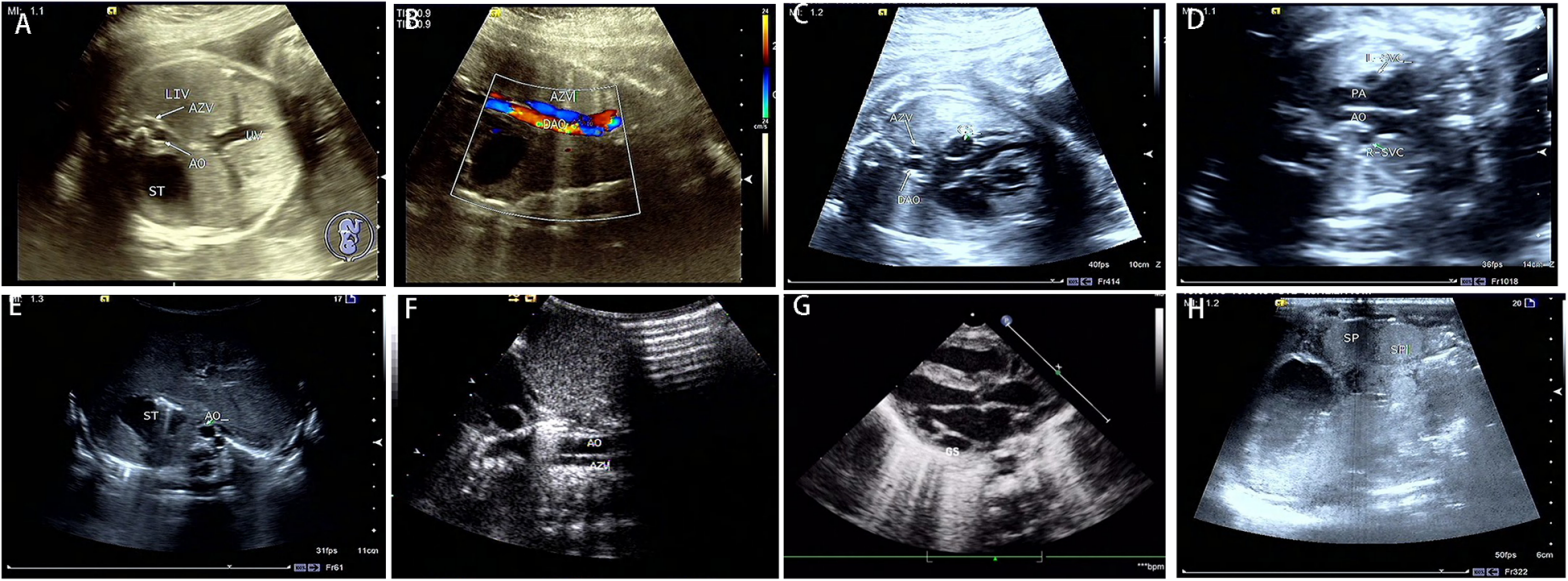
Prenatal and postnatal ultrasonography of LI fetuses. Prenatal sonography in the transverse section of the abdomen (A–D). (A) absence of a hepatic segment of IVC with dilated azygos; ST gastric vesicle, AO, abdominal aorta, AV azygos vein, and UV umbilical vein. (B) the parallel arrangement of the azygos vein and descending aorta. DAO, descending aorta. (C) the dilatation of coronary sinus. CS, coronary sinus. (D) double superior vena cava, L-SVC, left superior vena cava; R-SVC, right superior vena cava; PA, pulmonary artery. Postpartum sonograms (E–H): the images by postpartum sonography were consistent with prenatal ones.
